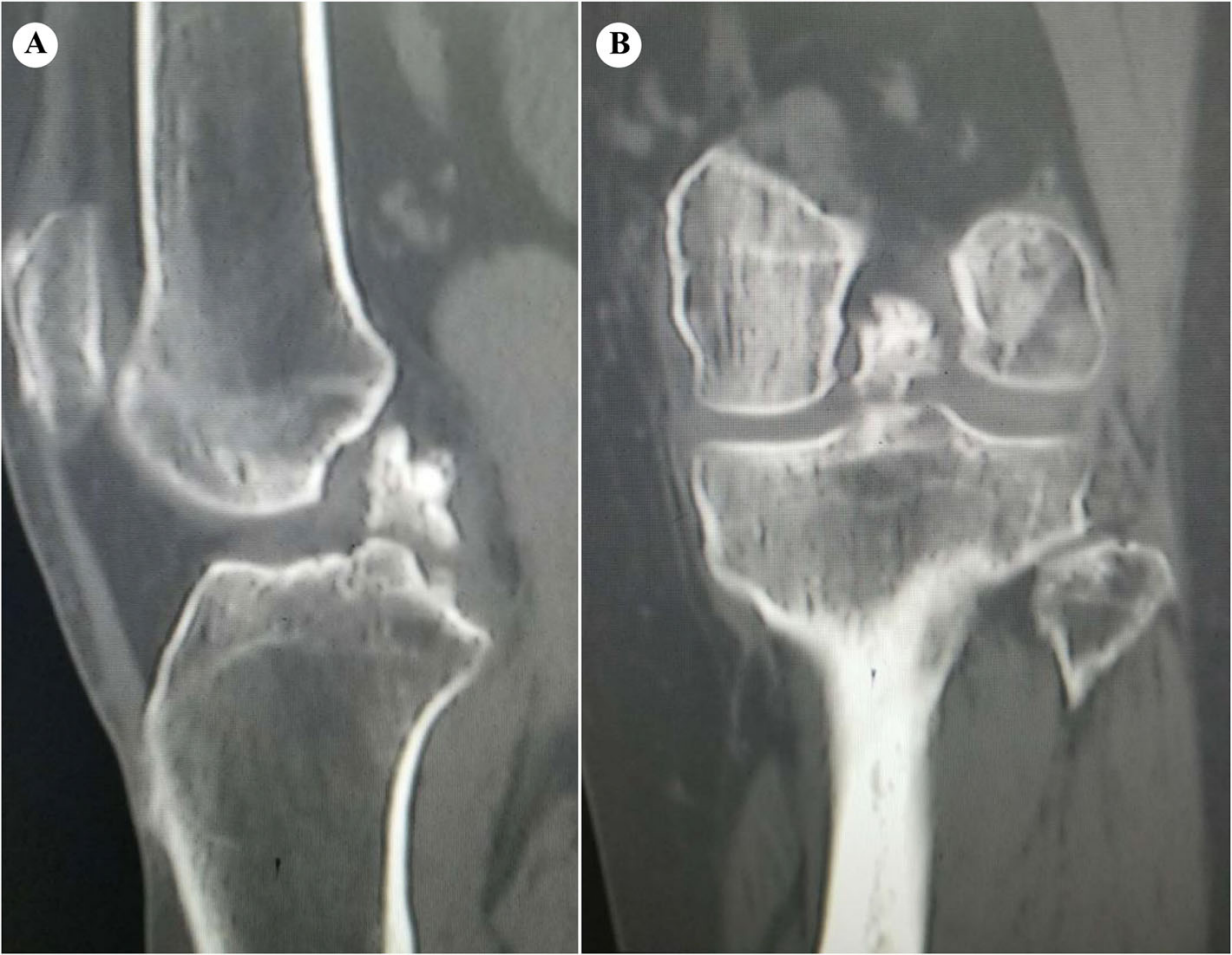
High-density mass is seen in the PCL by multi-planar reconstruction CT
